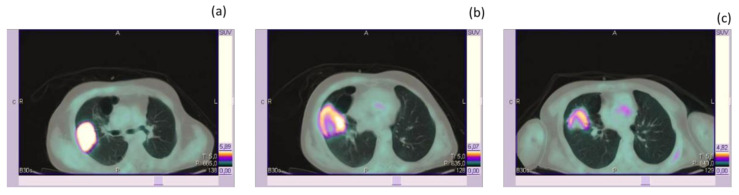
A representative example of metabolic response in patients with advanced NSCLC treated with Nivolumab. Baseline [^18^F]FDG PET/CT (a) shows a right parascissural lung lesion (dd max 7 cm; SUVmax 12). Only a mild reduction in lesion size was highlighted at the first response evaluation (2 months after therapy; b); however, a more marked metabolic reduction was already evident (SUVmax 6). Of note, a metabolic active volume reduction was even more evident than a SUVmax reduction as a central photopenic area was evident after treatment. The first metabolic response was able to predict the patient’s response evolution, as after a further two months, both lesion size and metabolism were further decreased (SUVmax 4, c). NSCLC: Non Small Cell Lung Cancer.
